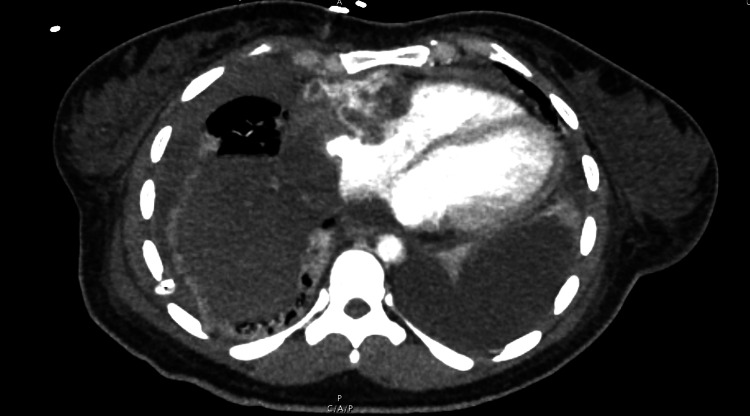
Chest CT showing large mass extending circumferentially into the pericardium, cardiac wall, and chambers
